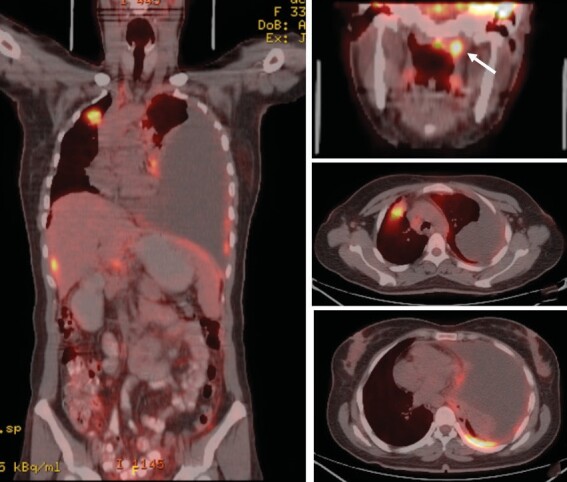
Figure 2. An increased FDG uptake in the mass lesion
